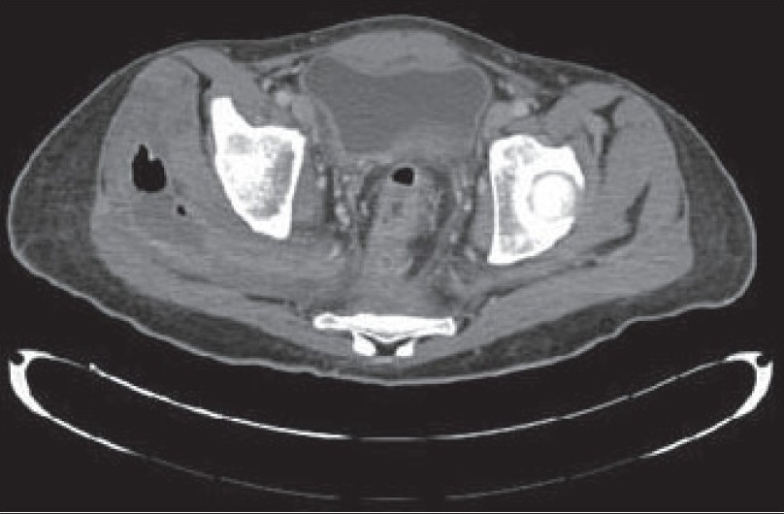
CT scans of the abdomen and pelvis
